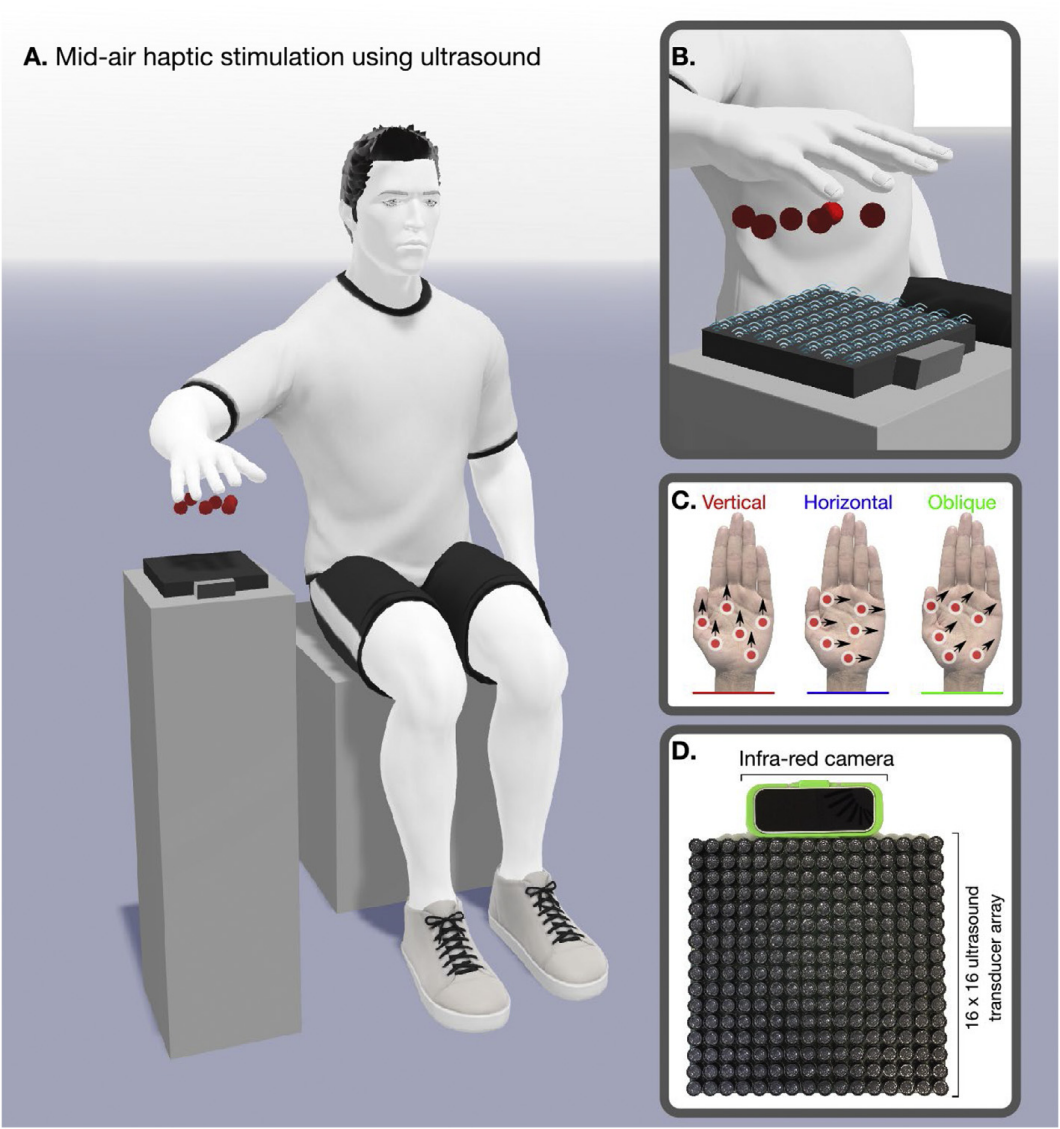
Overview of mid-air tactile experimental setup. A. Participants were seated with their hands above an array of ultrasound actuators and a infra-red camera. B. The combination of real time hand tracking and focused ultrasound can project discrete points onto the user's unadorned hand (Carter et al., 2013). C. Users experienced a series of moving dot stimuli (Fig. 2) in differing directions. D. Stimuli were delivered using an Ultrahaptics device (UltraLeap, Bristol).
